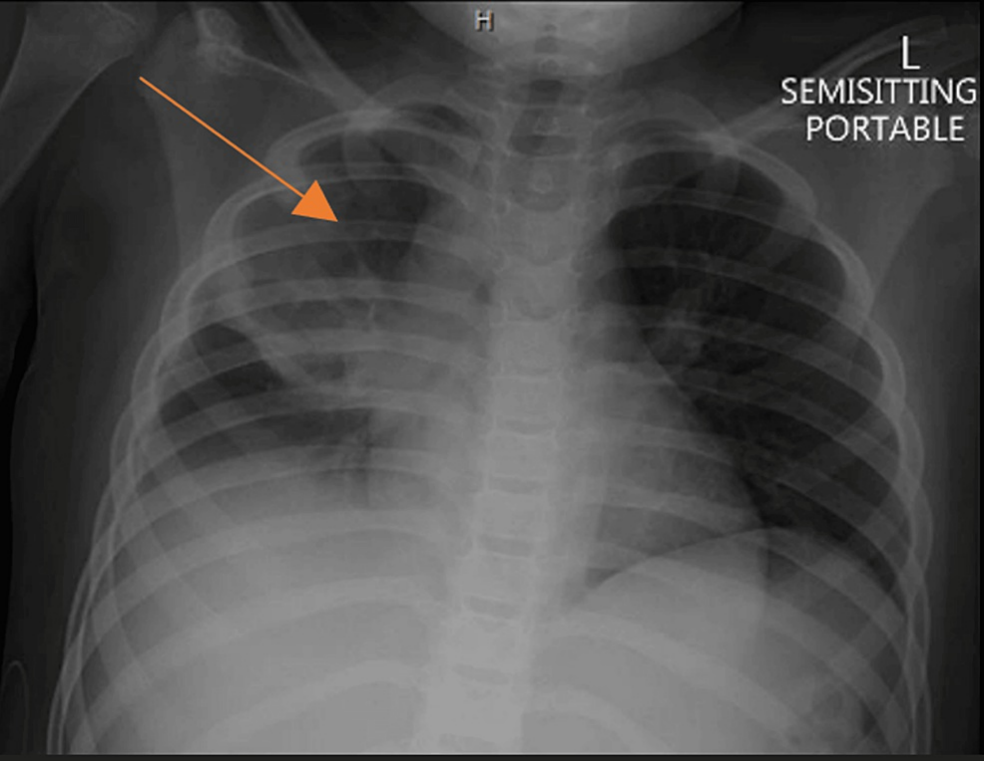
Chest X-ray. The improvement of the right pneumothorax with a stable upper zone opacity. The arrow pointing to the right upper zone opacity.
